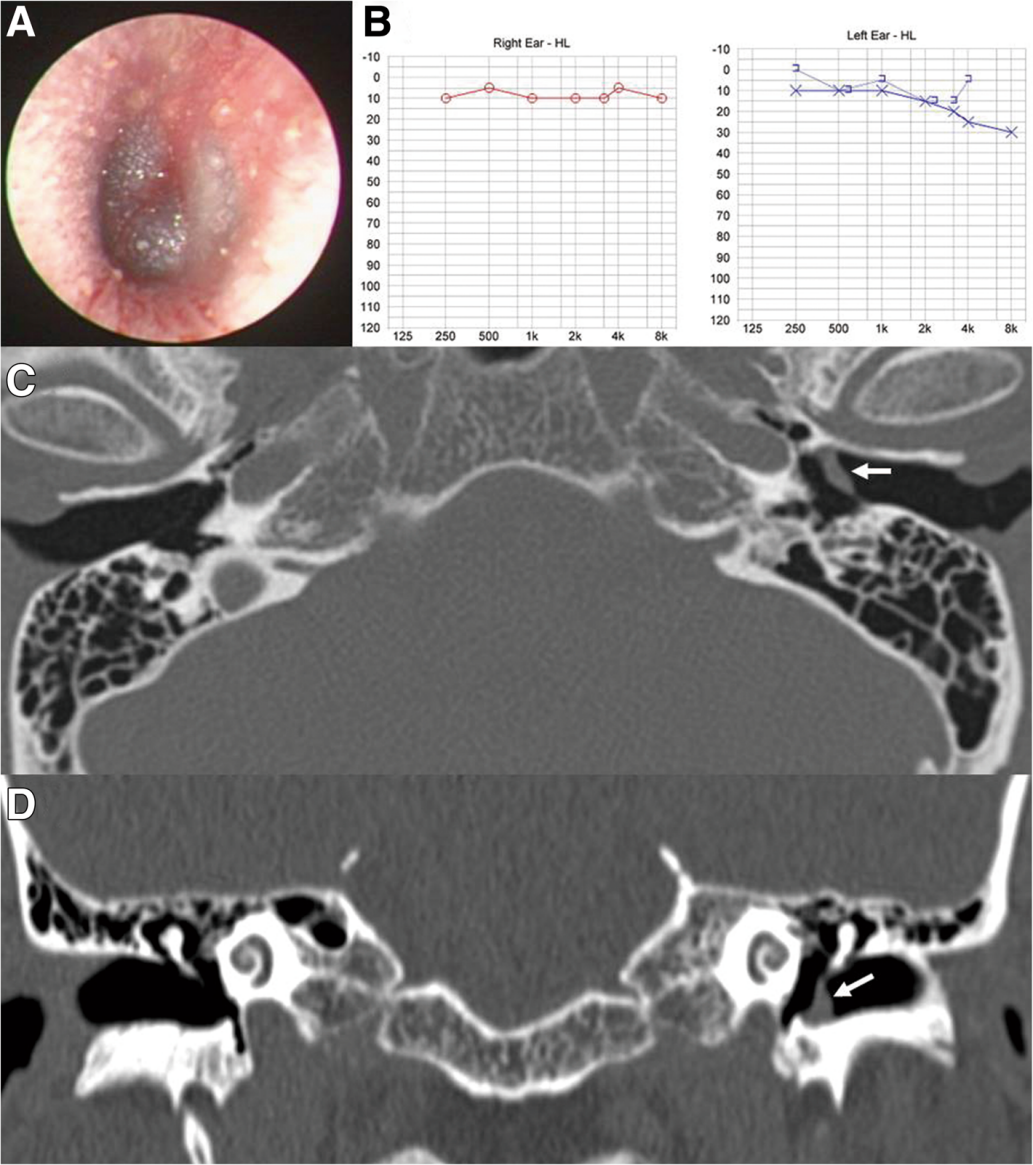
Otoendoscopic examination (a) of patient 3 showed bluish thickening of the anterior part of the intact left tympanic membrane (TM). Pure tone audiometry revealed mild conductive hearing loss on the left side (b), which was improved after 2 weeks (c). Temporal bone computed tomography revealed thickening of the left TM (white arrows) on axial (d) and coronal views (e), suggesting iTM hemorrhage
